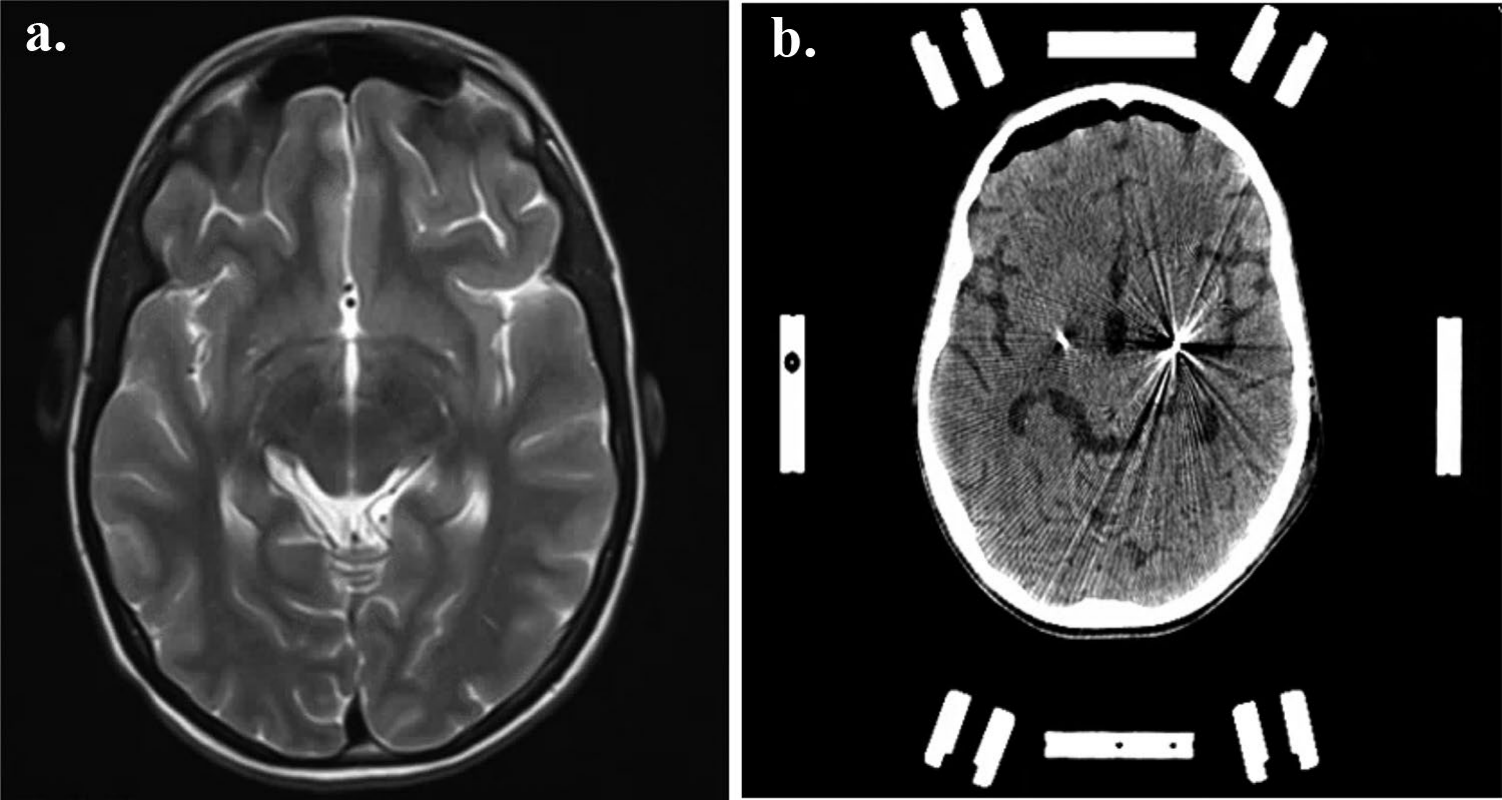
a MRI of the brain: T2-weighted axial image shows no abnormalities. b Postoperative stereotactic CT shows the positioning of the electrodes in the posteroventral lateral globus pallidus internus
